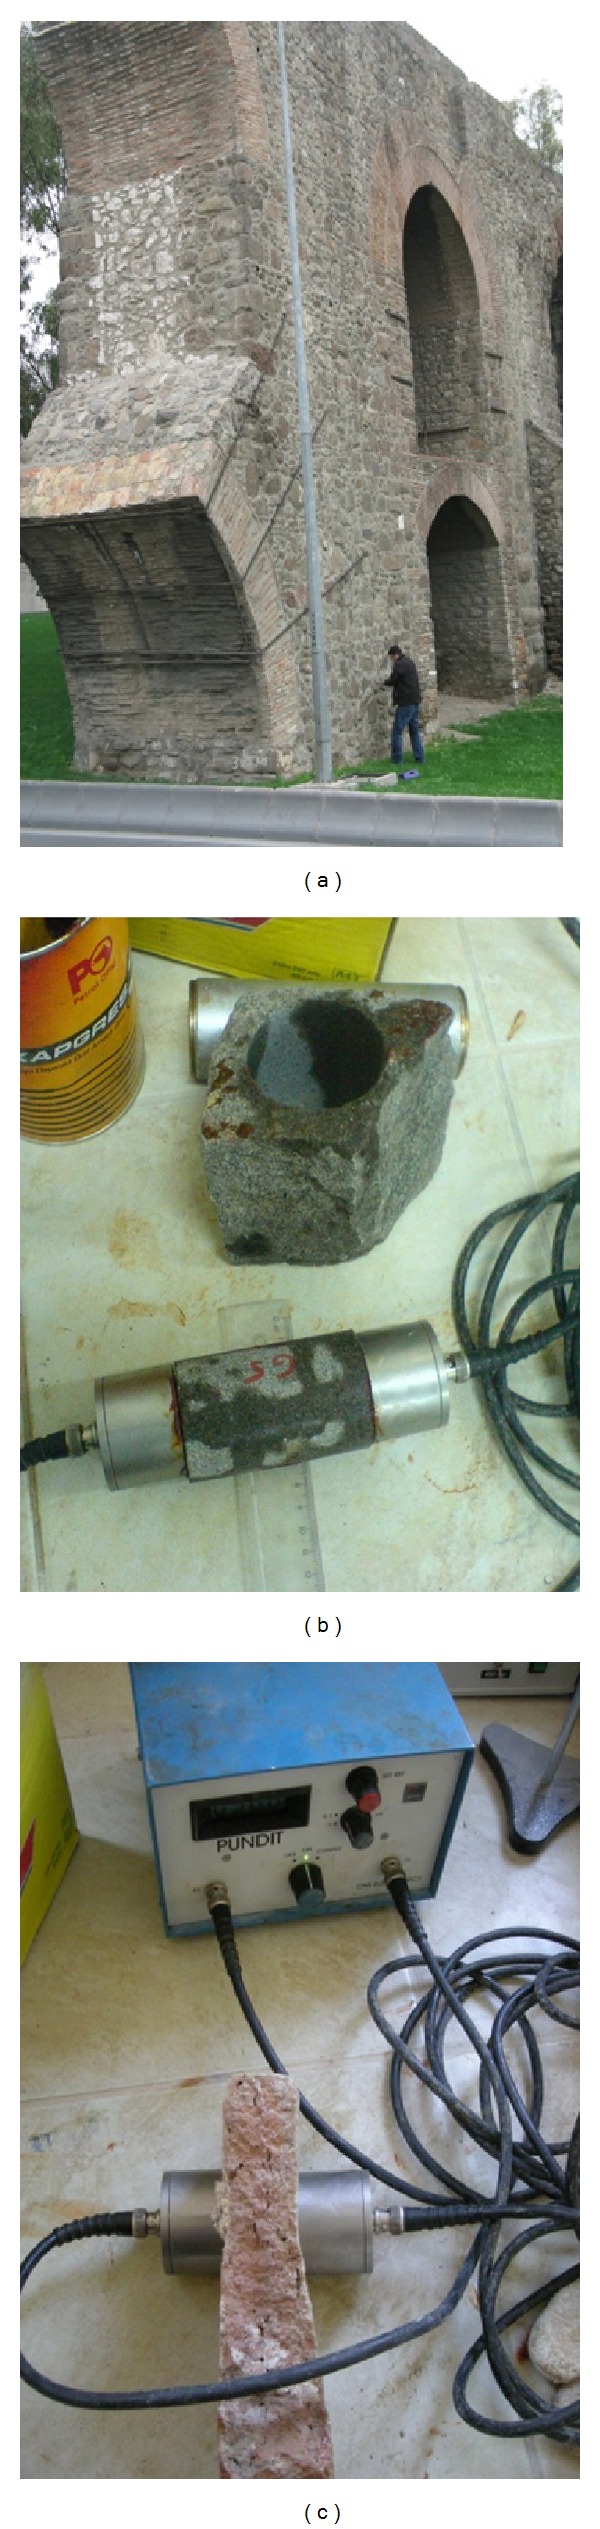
In situ* Schmidt test and ultrasonic wave velocity test on stone and brick.
